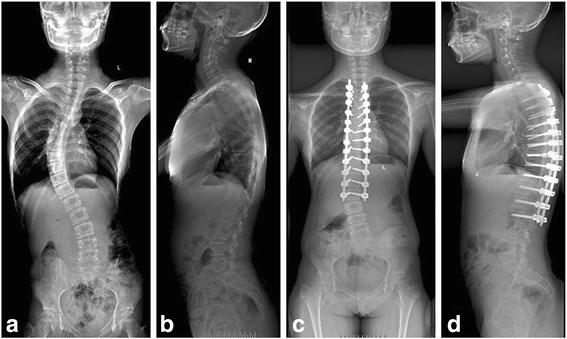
A high-density pedicle screw construct was used. Preoperative standing anteroposterior (a) and lateral radiographs (b). Final follow-up standing anteroposterior (c) and lateral radiographs (d)
